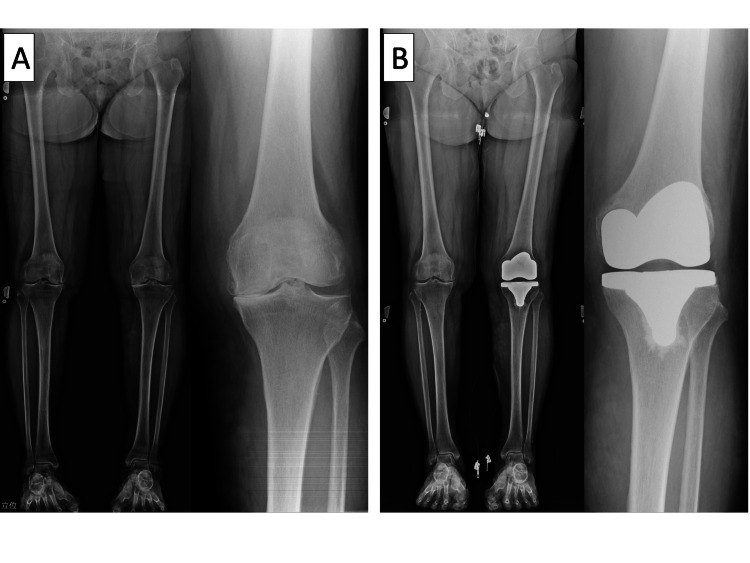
A) Standing lower limb X-rays before surgery and B) standing lower limb X-rays after surgery
